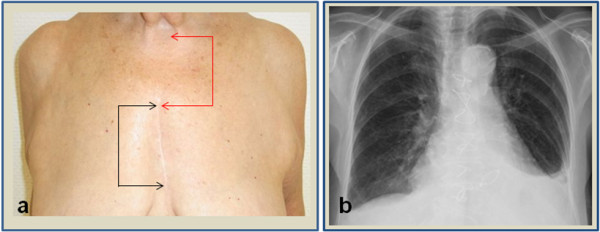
Position of the scar. (a) Scar (black arrows) position approximately 5 cm below jugulum (red arrows). (b) chest x-ray showing sclerotic aorta and adaptation of the sternum with standard steel wires.
